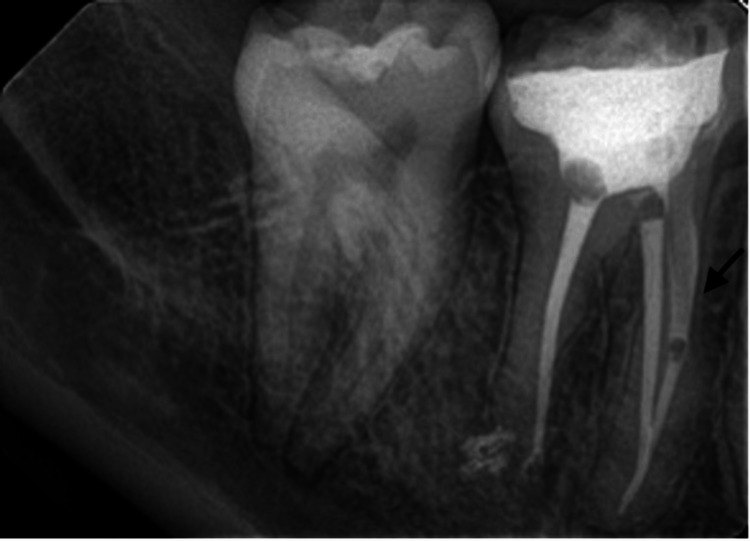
Periapical radiograph after placement of the fiber post and core build-up.
